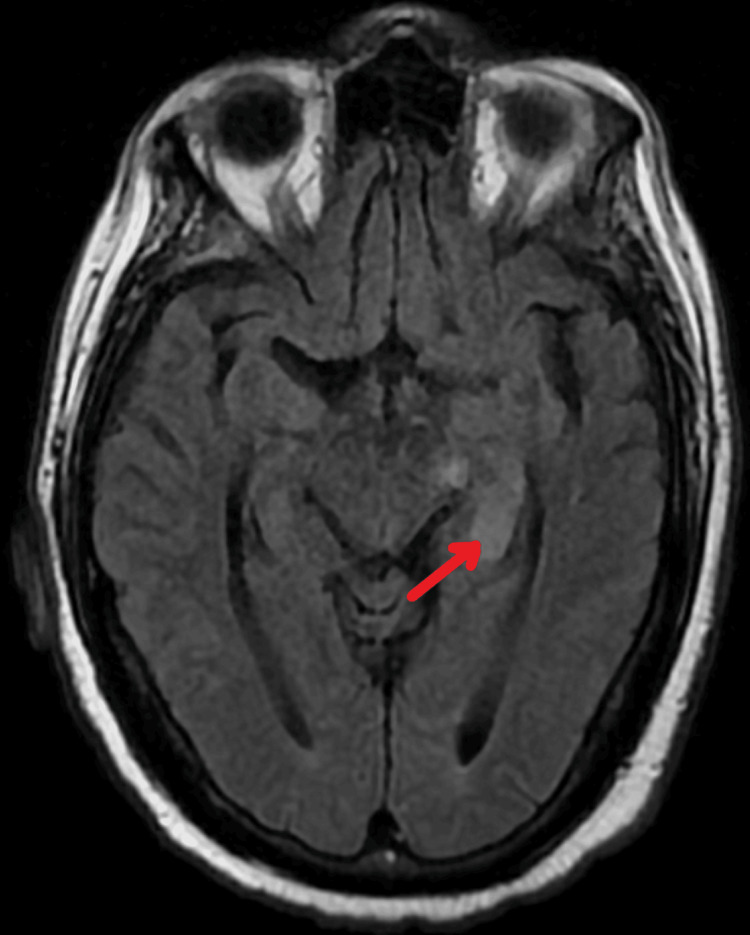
T2-weighted MRI brain. The red arrow indicates the area of subacute infarct in the left temporal lobe of the brain.
